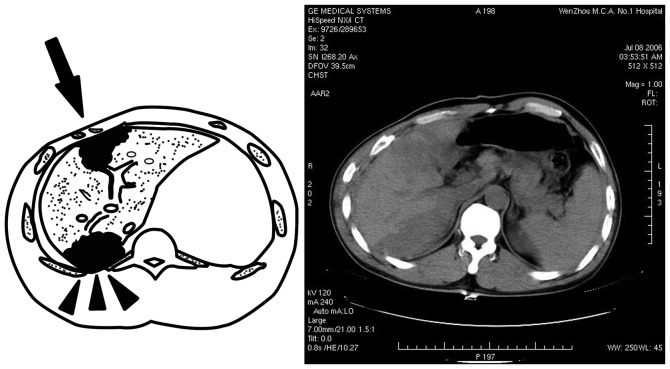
Compression injury of the right lobe of the liver. When the upper right quadrant is under compression in an anterior-posterior manner, the liver is crushed between the anterior and posterior walls of the rib cage (black arrow and black arrowheads, respectively) which lacerates the posterior and anterior sides of the liver at the same time. CT film shows a hepatic injury caused in this way by a traffic accident.
